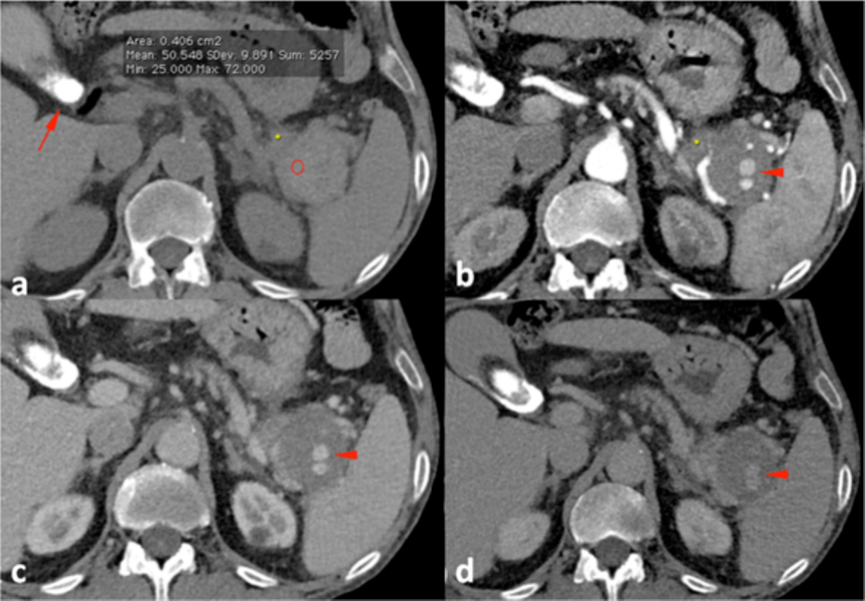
Initial radiology findings. (a) Non-enhanced computed tomography showing a thin, dense imbibition of fat around the pancreatic tail (asterisk) near a 5.1cm pseudocyst with hyper-dense content and a gallstone inside the gallbladder (arrow). (b-d) Contrast-enhanced computed tomography shows a pseudoaneurysm arising from a splenic artery branch inside the pseudocyst.
